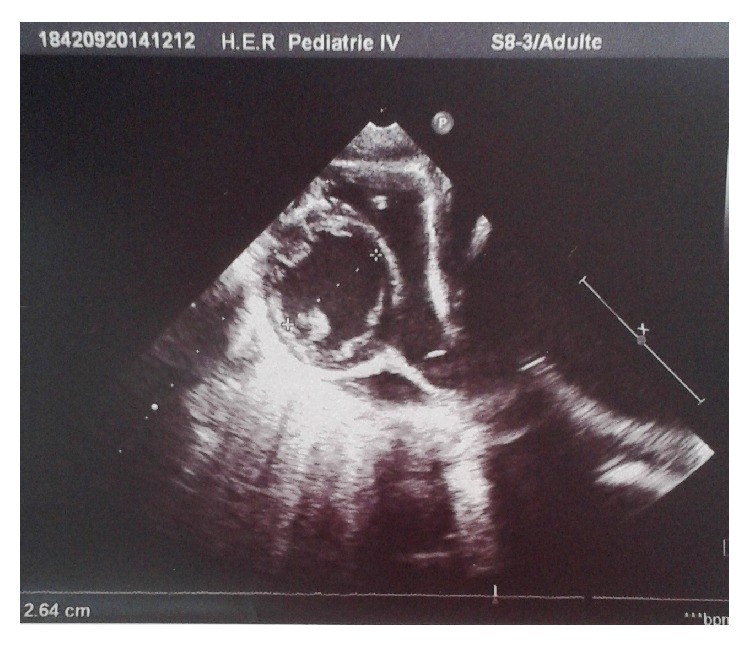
Echocardiography showing a 15 cm heart mass compressing the two atria.
